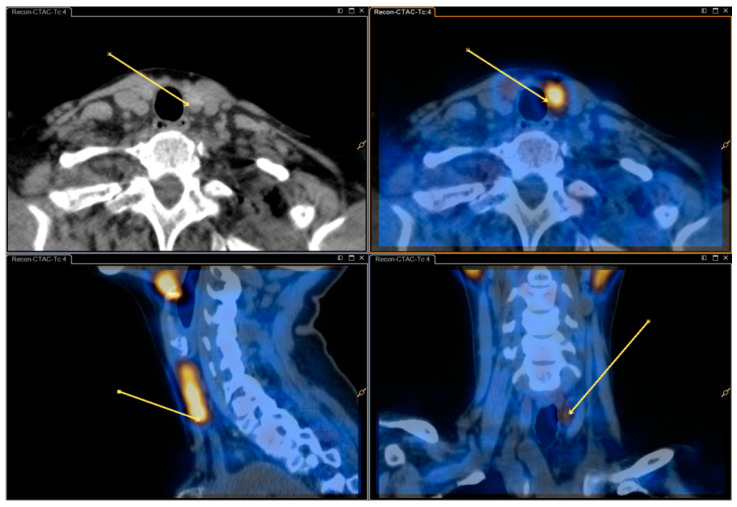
Single isotope SPECT/CT image (Tc-99m-sestamibi) with and without fusion—same patient as in Figure 1.
